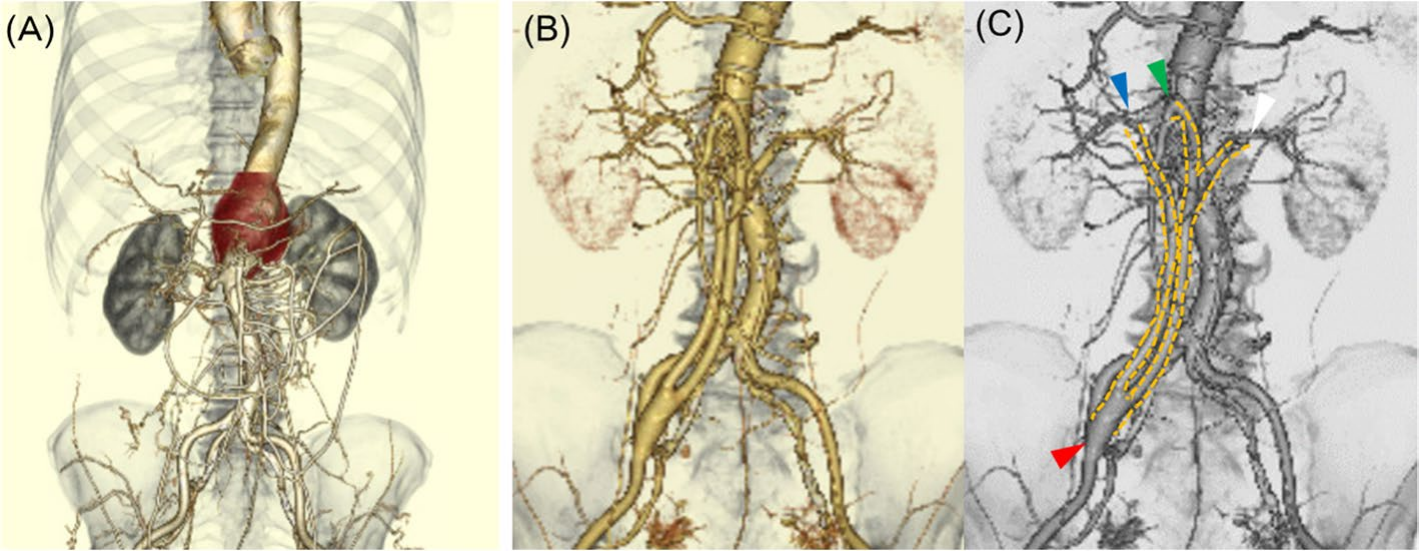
A Preoperative 3-dimensional CT angiography before the initial surgery 10 years ago of supra-renal abdominal aortic aneurysm which was 60 mm in diameter. The celiac artery originated from the aneurysm and occluded. B, C After initial surgery (renovisceral debranching and aortic stent-graft placement) in 2 different colors, the proximal side of 12 × 6 mm bifurcated GORE-TEX Vascular Graft (W. L. Gore & Associates, Inc. Flagstaff, AZ, USA) (yellow dotted line) was anastomosed to the right common iliac artery (red arrowhead), distal end of bifurcated graft was anastomosed to the right (blue arrowhead), and left renal artery (white arrowhead), respectively. A straight graft (green arrowhead) was placed between the superior mesenteric artery and the bifurcated graft toward the left renal artery. Six days after vascular graft placement, we placed a 180-mm length of INOUE STENTGRAFT between descending aorta and the abdominal aorta which is the level of Th11-L4. The proximal diameter of the stent graft was 30 mm, and the distal diameter was 20 mm
